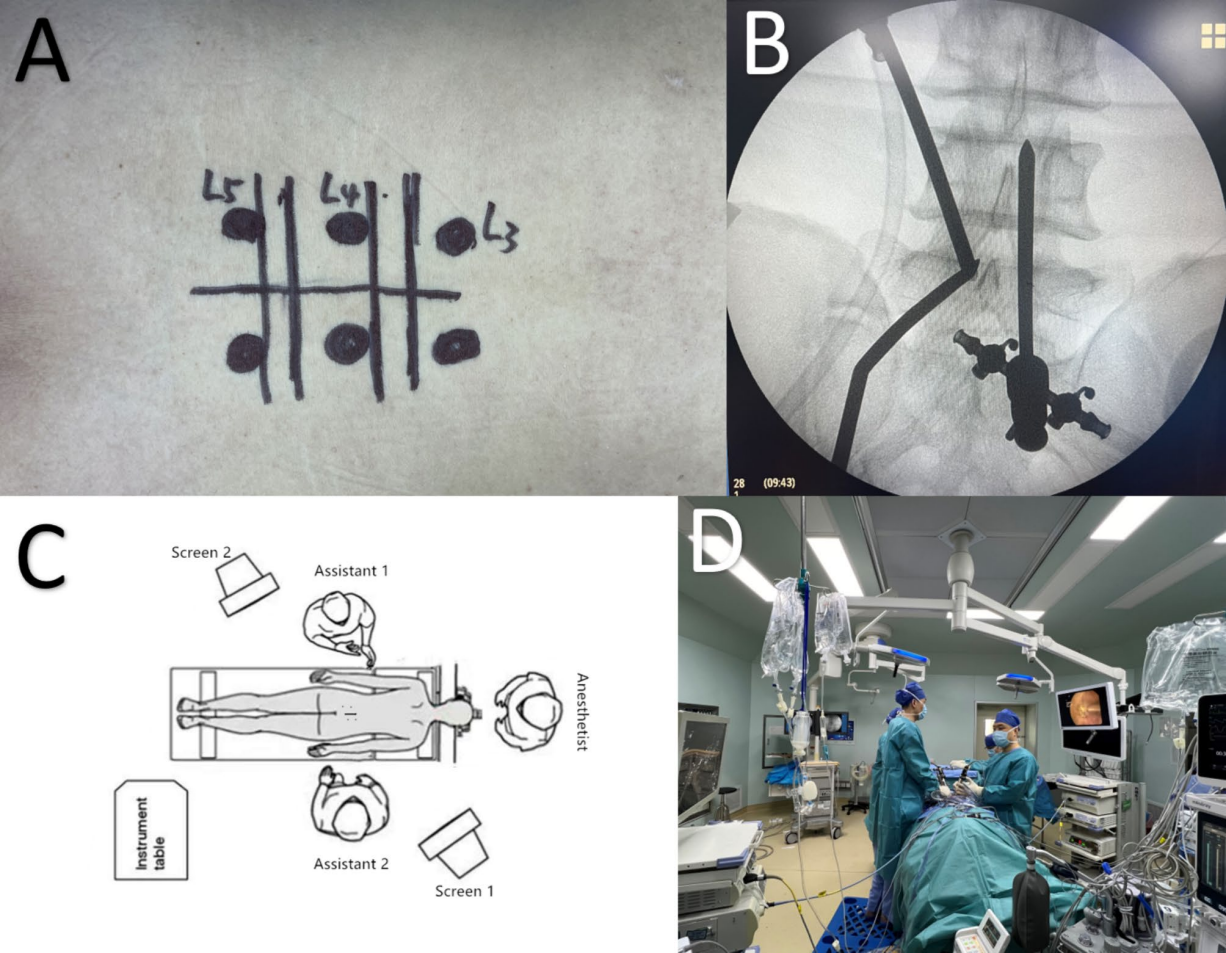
Case 1; Male, 54 years old, lumbar spinal stenosis L3 ~ 5, L3/4 right approach decompression, L4/5 left approach decompression, bilateral simultaneous. A: L3/4 preoperative CT, B: L4/5 preoperative CT, C: preoperative CT reconstruction; D: L3/4 postoperative CT, E: L4/5 postoperative CT, F postoperative CT reconstruction showed that the right L3/4 and the left L4/5 laminae were missing.
